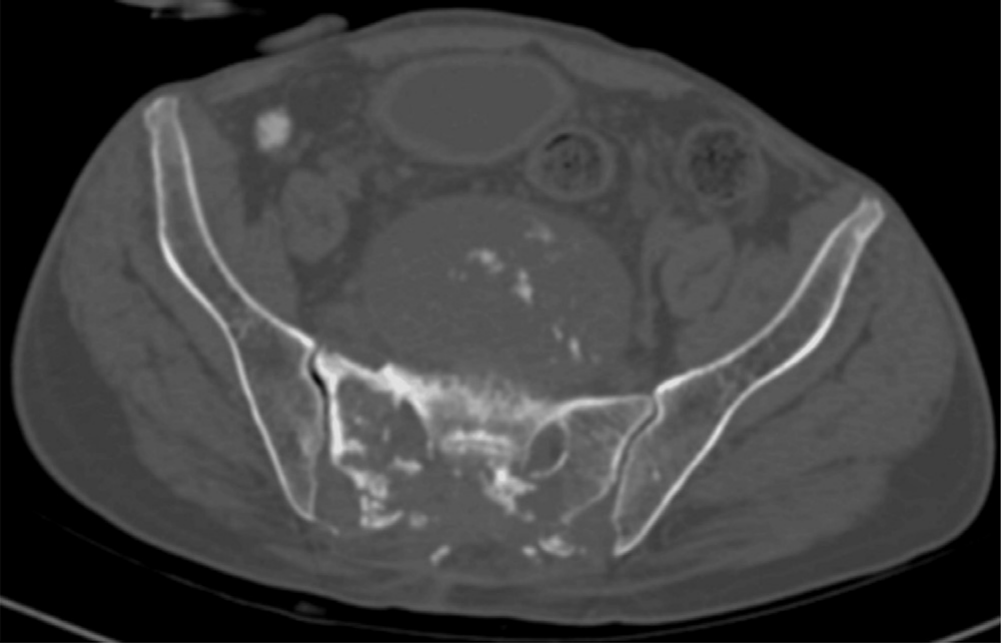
CT axial section bone window showing destruction of sacrum caused by the lesion.
